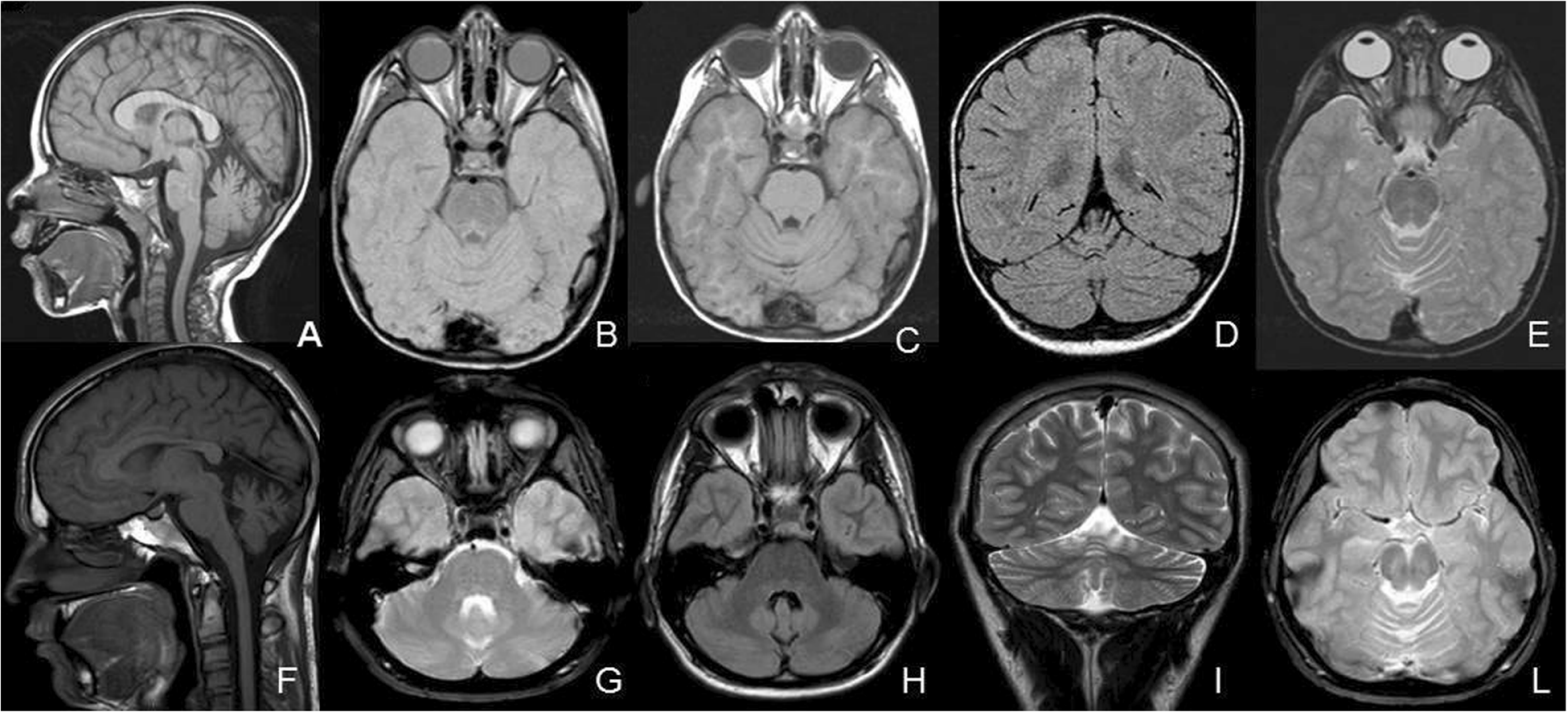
Brain MRI at 4 (first row) and18 (second row) years of age, showing stable superior vermis atrophy and reduced volume of the optic nerves. a and f: sagittal T1 sequences, b and g: axial T2 sequences, c and h: longitudinal FLAIR sequences, d: coronal FLAIR and i: coronal T2 sequences, e and l: longitudinal T2 sequences
